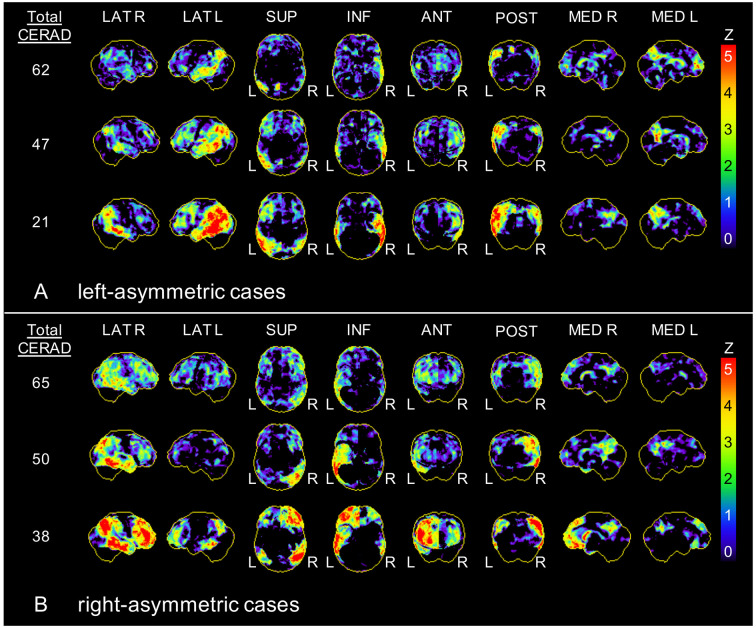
Exemplary asymmetric hypometabolism patterns. Three-dimensional stereotactic surface projections (*Z*-score maps) of patients with (A) left-asymmetric and (B) right-asymmetric hypometabolism in F-18-fluorodeoxyglucose positron emission tomography (FDG-PET). CERAD, Consortium to establish a registry for Alzheimer’s disease; R, right; L, left; LAT, lateral; SUP, superior; INF, inferior; ANT, anterior; POST, posterior; MED, medial.
